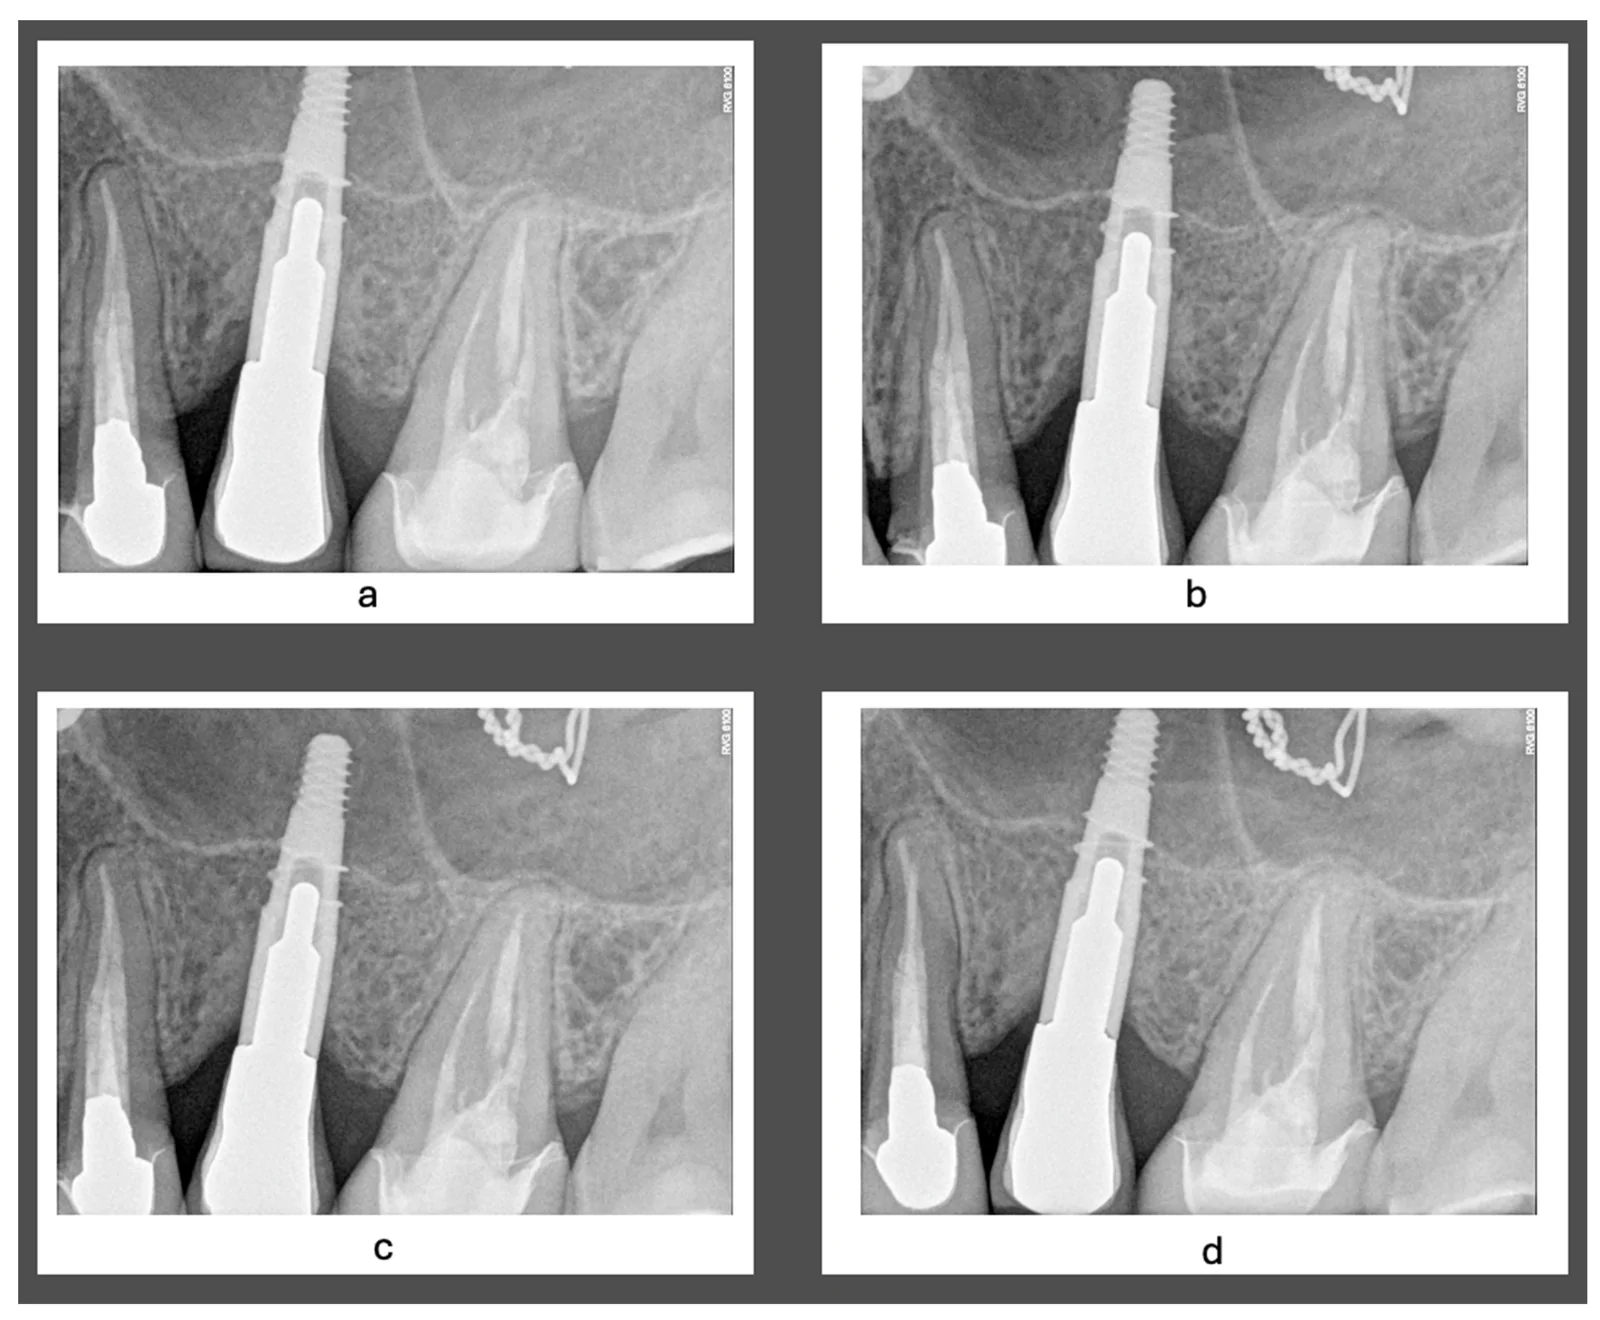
Intraoral radiographs acquired using an RVG 6100 digital sensor (Carestream Dental; resolution > 20 lp/mm) at baseline (a), 3 weeks (b), 6 months (c), and 12 months (d).
